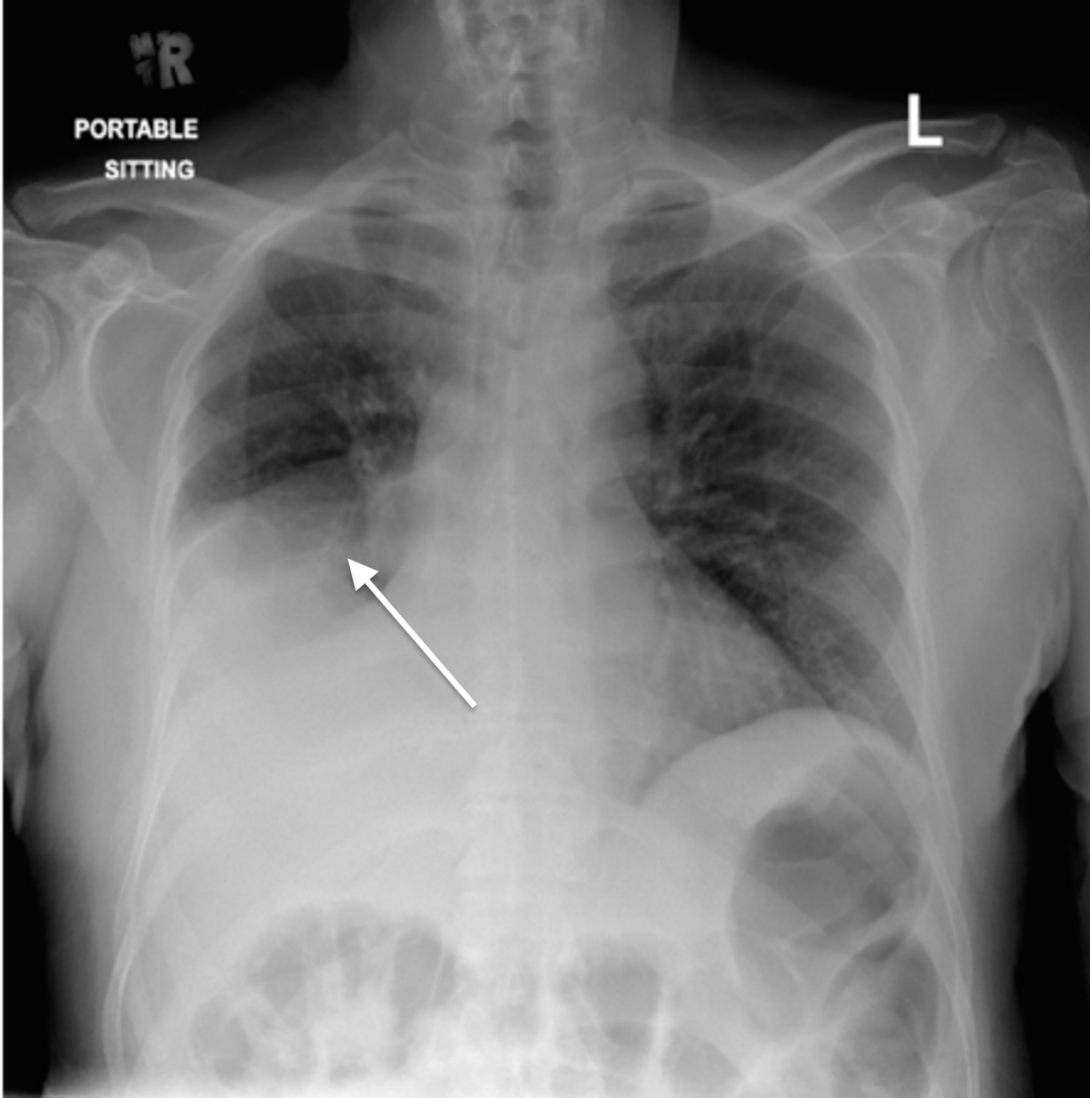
Chest X-ray on presentation
